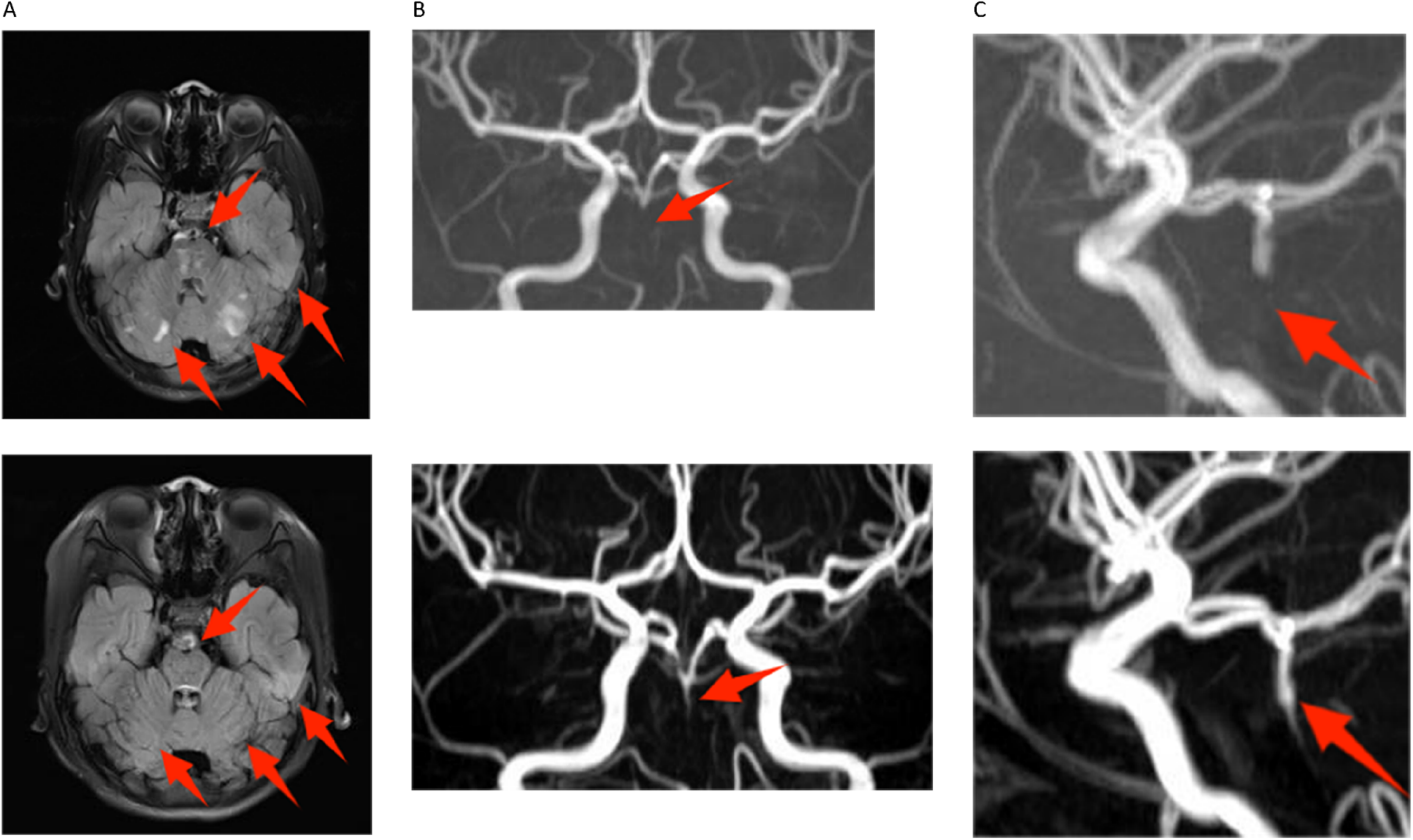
Magnetic resonance imaging in both hospitalizations. The image above is from 2021, and below is from 2023. (A) Compared to the 2021 image, the fluid-attenuated inversion recovery (FLAIR) images of transverse relaxation time (T2) weighted from 2023 showed multiple old and new abnormal signals in bilateral pons and cerebellar hemispheres. Notably, the size of the old lesion has decreased (indicated by red arrows). (B,C) The proximal part of the basilar artery was not visualized on digital subtraction angiography in either the 2021 or 2023 images. However, the middle and distal segments were partially visualized in the 2023 image (indicated by red arrows).
